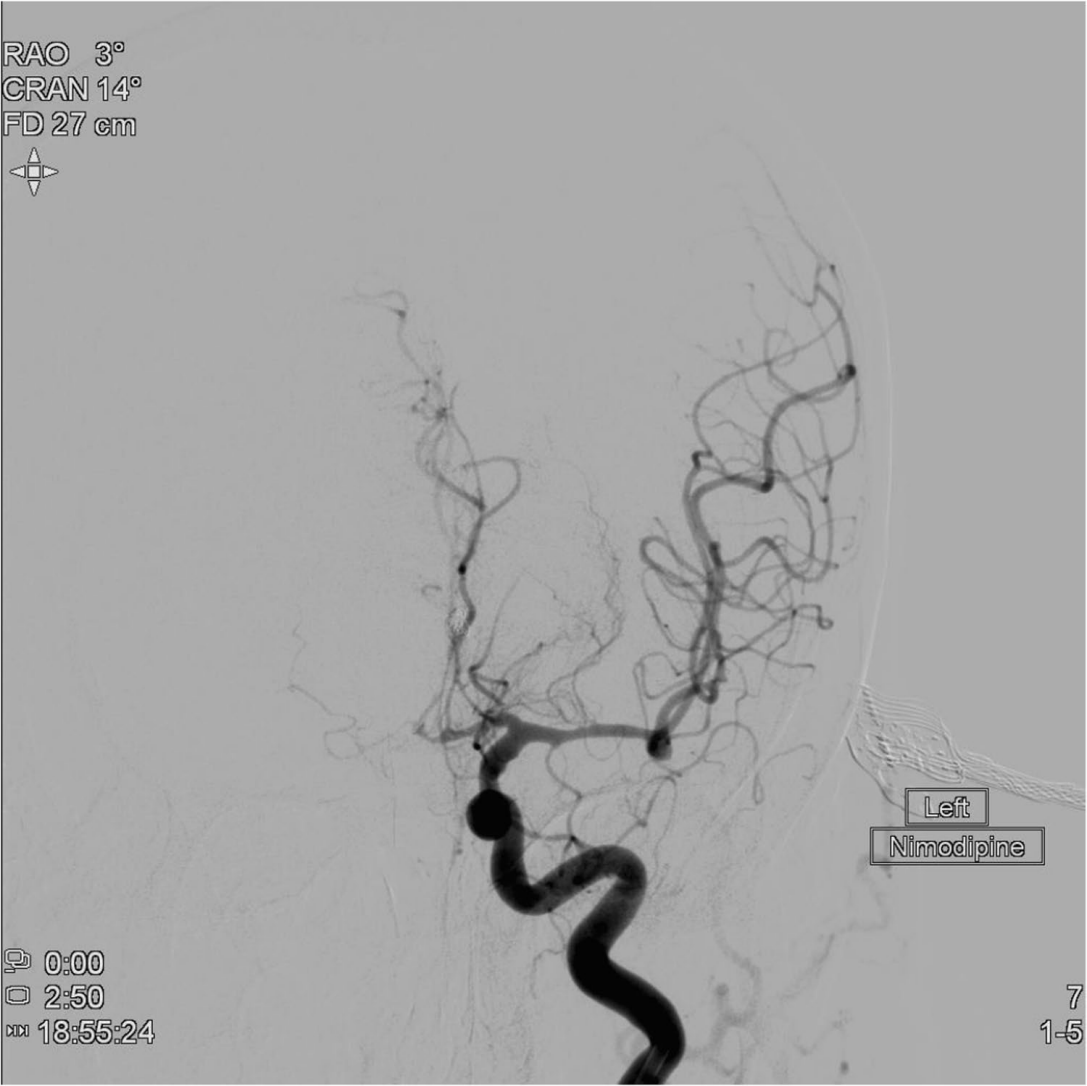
AP radiograph from digital subtraction angiography (left internal carotid artery injection), performed for patient with subarachnoid haemorrhage and radiographic vasospasm
